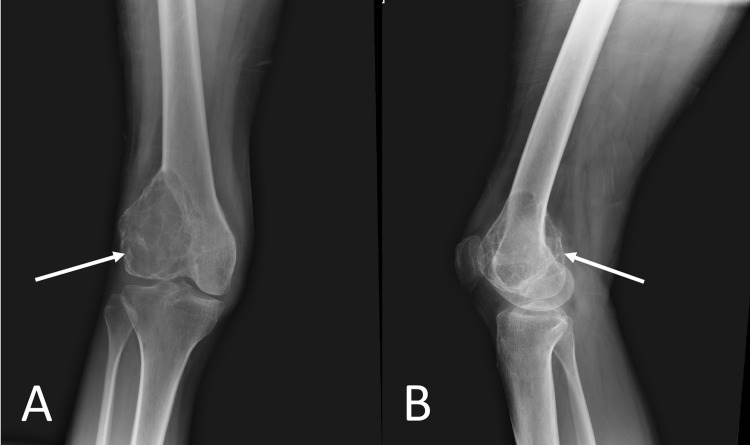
Plain radiograph of left knee joint Subarticular, eccentric, lytic lesion, with thinning of the cortex and no peripheral sclerosis in the lateral femoral condyle shown by the white arrow. (A) Anteroposterior view. (B) Lateral view.
